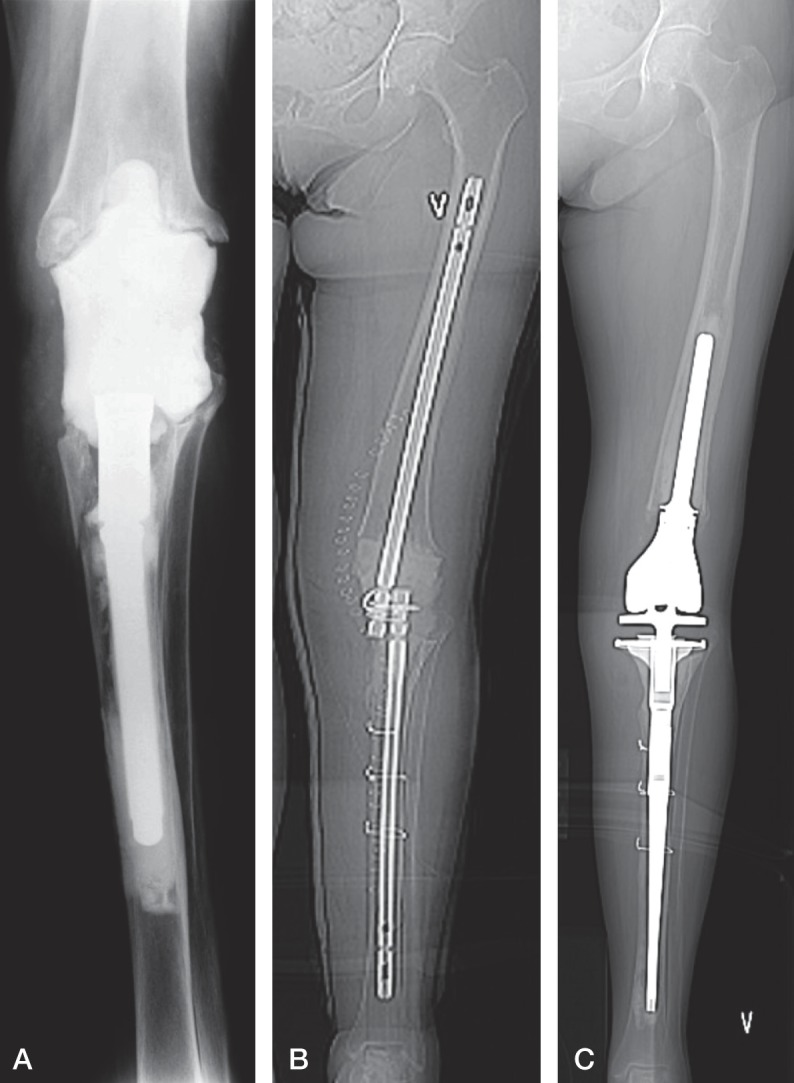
A 79-year-old woman with candida ostitis, who had the least bone loss on the femoral side, and the most bone loss on the tibial side. A. Status at referral with a cement spacer and the tibial stem still well-fixed to the bone. B. After revision, including removal of the tibial stem and insertion of an intramedullary spacer made by 2 retrograde nails connected with wires plus bone cement. C. X-ray after removal of the spacer and insertion of a tumor prosthesis (C).
